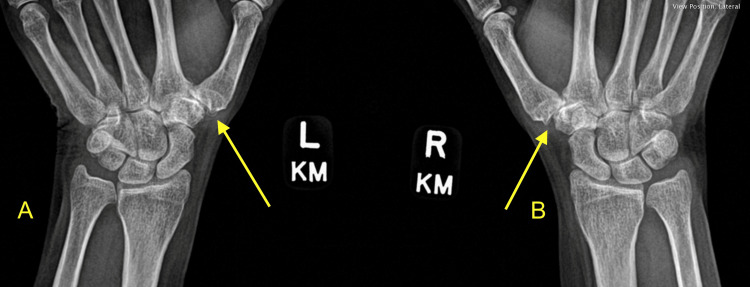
X-ray displaying preoperative degenerative changes of bilateral CMC joints. (A) Left CMC joint arthritis with arrow indicating the joint space narrowing, osteophyte formation, and joint subluxation. (B) Right CMC joint arthritis with arrow indicating joint space narrowing, osteophyte formation, and joint subluxation. CMC: carpometacarpal
